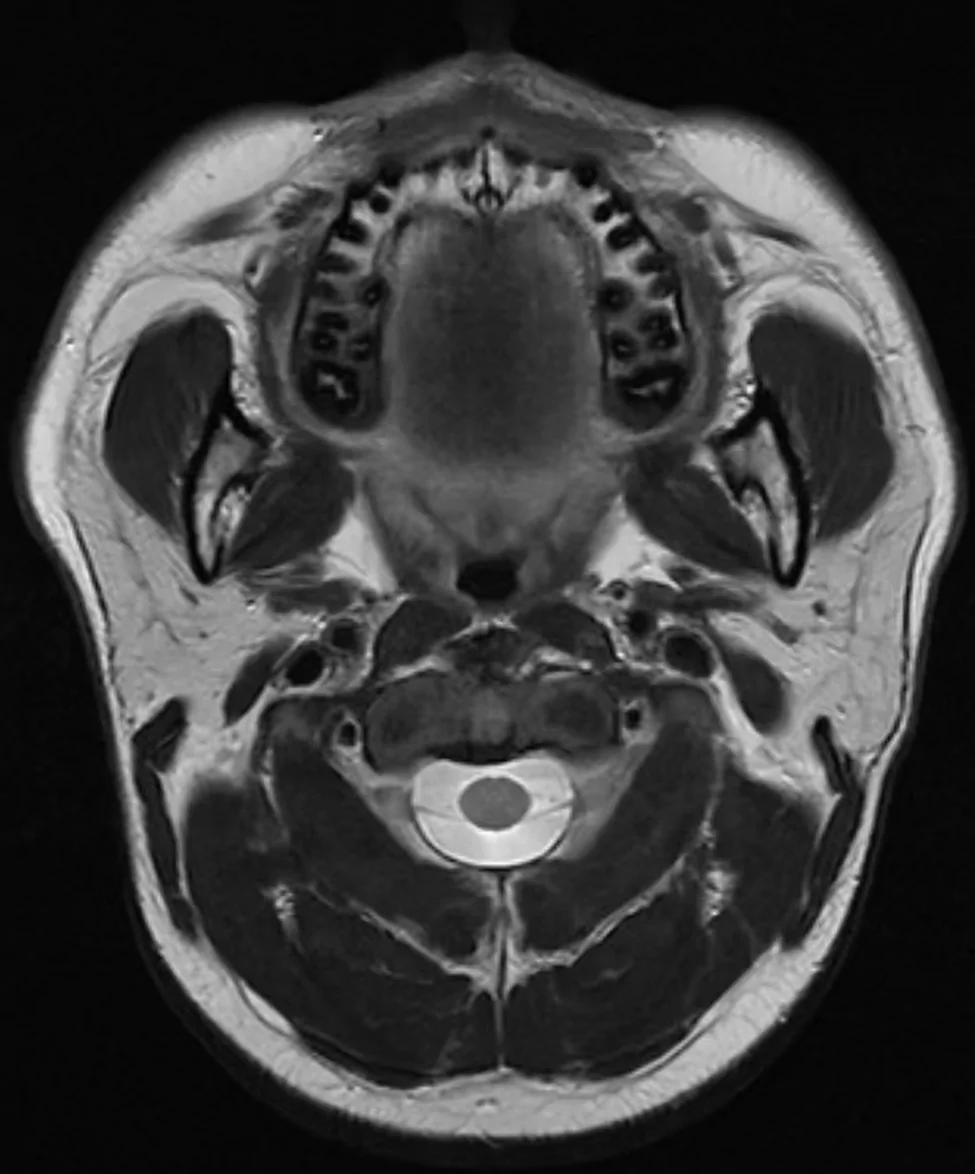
MRI images obtained during the patient’s remission period revealed no significant abnormalities in brain and orbital
